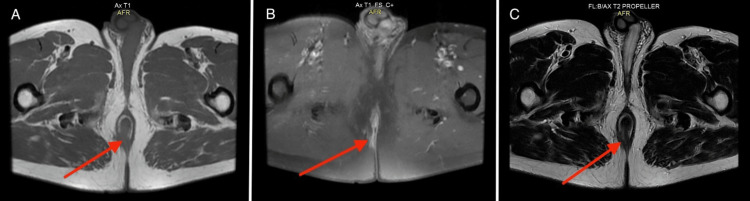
Pelvic MRI with contrast agent injection (A) Axial section T1 sequence (B) Axial section T1 sequence with fat saturation (C) Axial section T2 sequence. The images are revealing a tumoral process (red arrows) in the perianal region. The parasagittal median has well-defined regular contours, showing Hyposignal T1 and Hypersignal T2, enhancing post-contrast injection.
